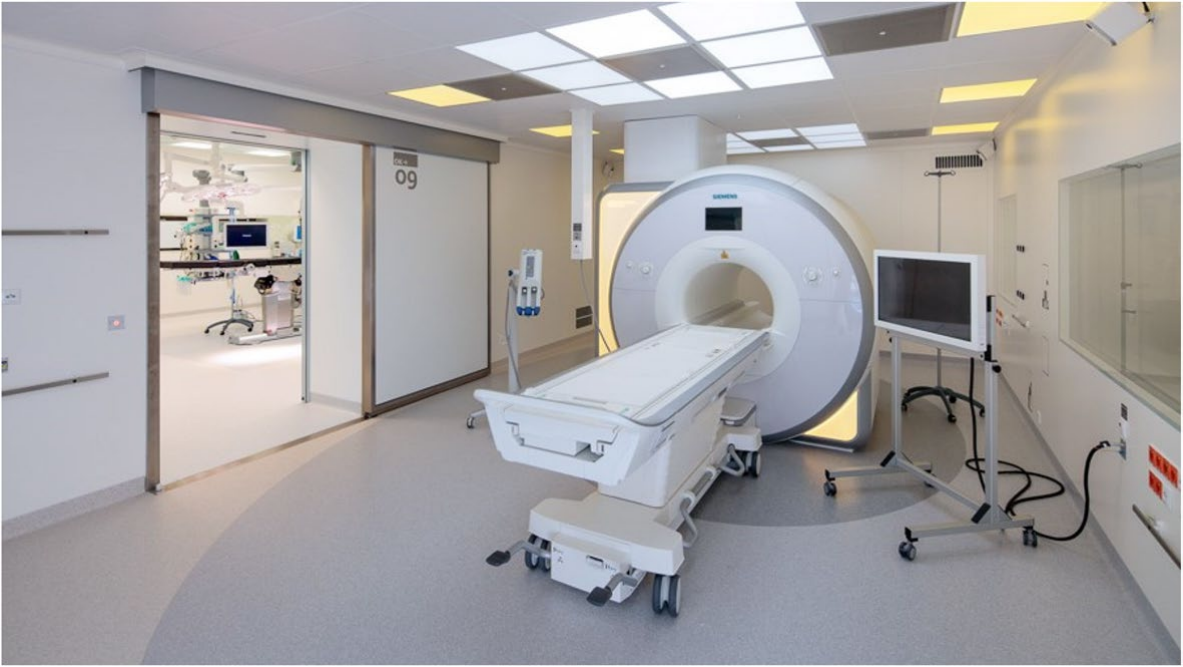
MRI scanner with dockable table and adjacent OR
